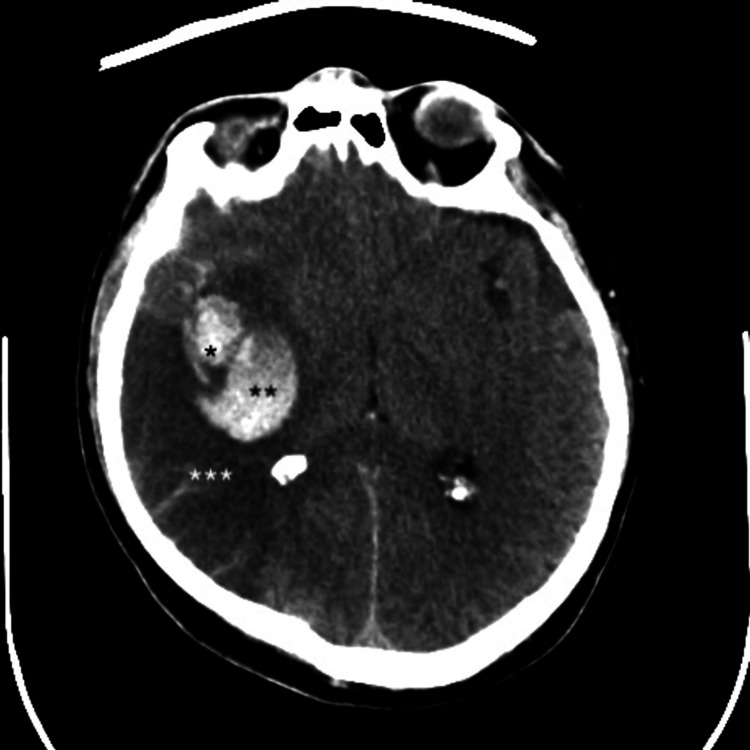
Axial brain CT scanner showing a subarachnoid hemorrhage associated with a right temporal hematoma, along with a right parieto-occipito-temporal junction ischemic stroke. *Partially thromboses M2M3 aneurism. Right temporal hematoma. *Ischemic lesion.
